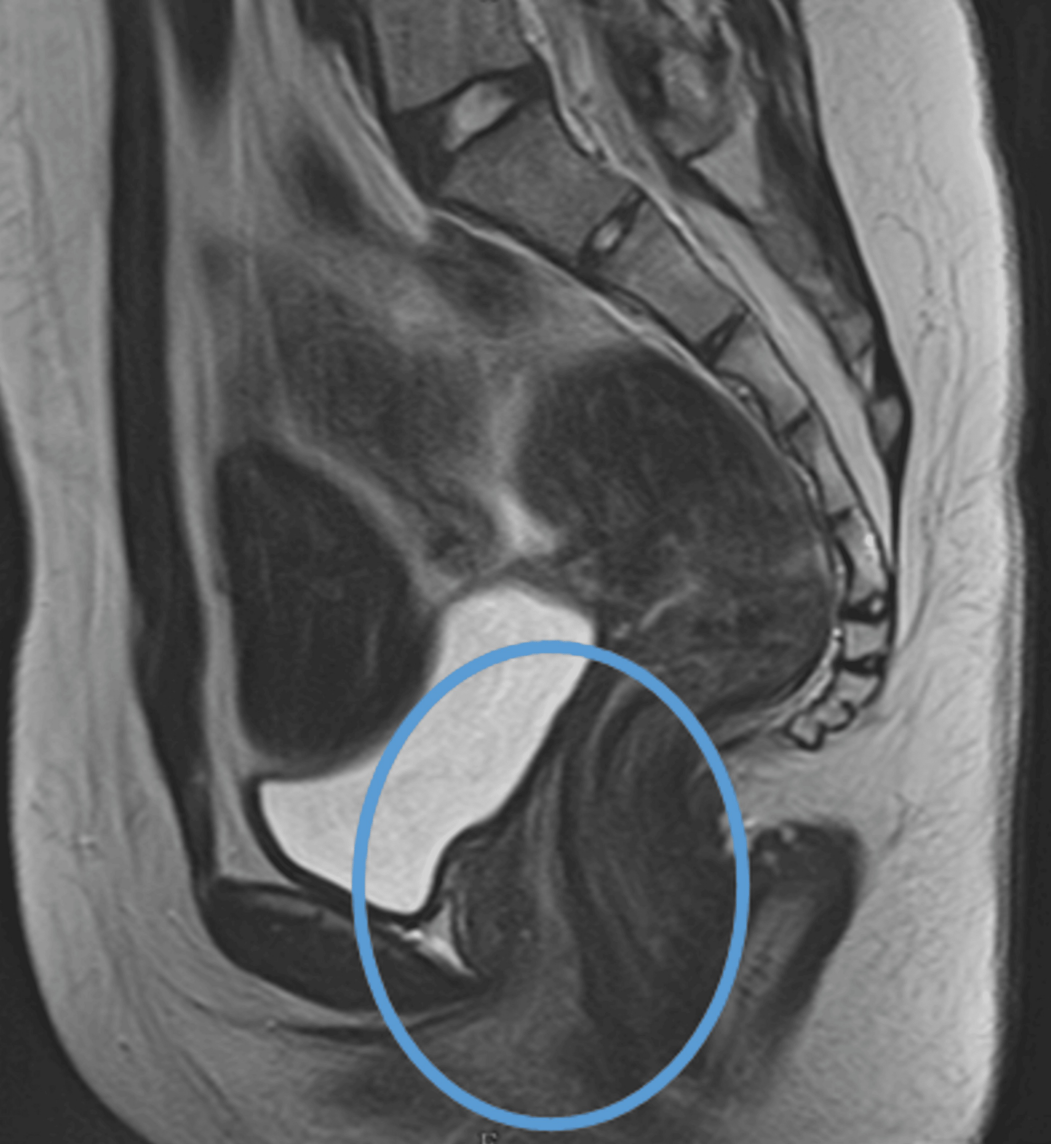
Pelvic magnetic resonance imaging: sagittal section. The circled area highlights the location where the uterus is absent and the vagina ends in a blind pouch.
